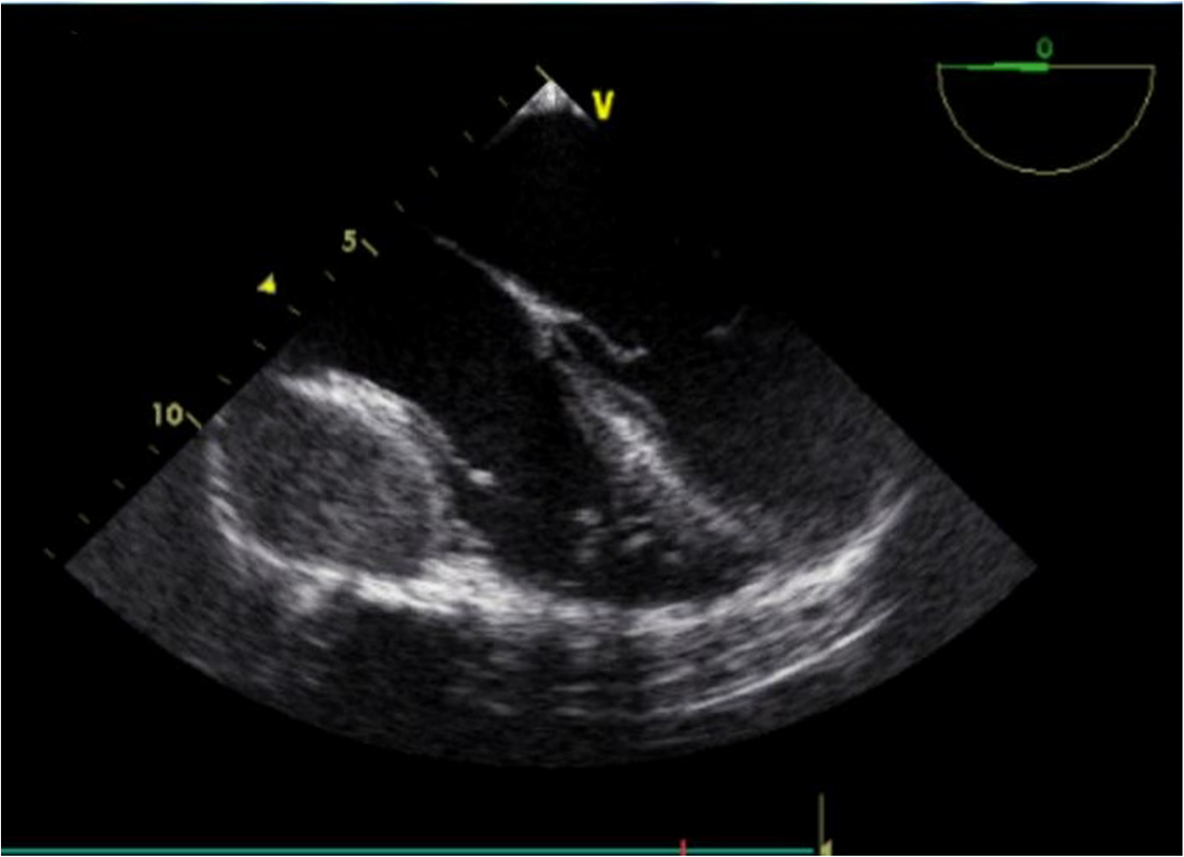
Bidimensionnal multiplane transoesophageal echocardiography: apical 4-chamber view incidence 0° showing mass in the pericarial mass around the right auriculo-ventricular junction.
